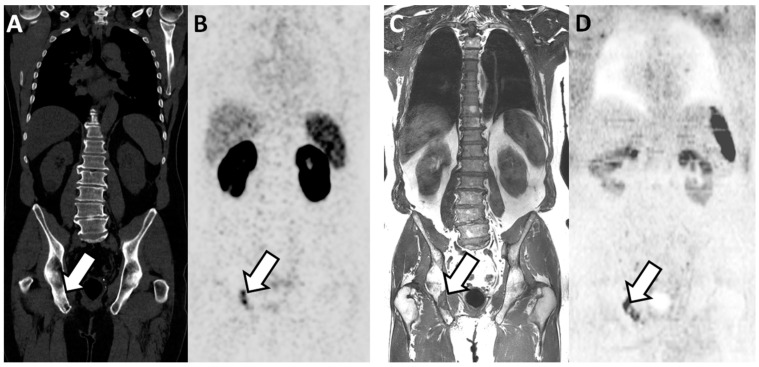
Concordant findings between PSMA PET/CT and WB-MRI/DWI: true positive observation of bone metastasis by WB-MRI/DWI in 65-year-old patient with ND prostate cancer at high risk for metastasis. (A,B) PSMA PET/CT: reformatted coronal CT (A) and PET (B) images show sclerotic lesion within the right ilio-ischiatic ramus with evident tracer uptake (arrows in (A,B)). (C,D) WB-MRI/DWI images: corresponding reformatted high resolution T1 (C) and diffusion-weighted (D) MRI images show bone lesion of low signal intensity on T1 and high signal intensity on MR images (inverted grey scale in the figure) within the right ilio-ischiatic ramus. The best valuable comparator confirmed the metastatic nature of the lesion.
